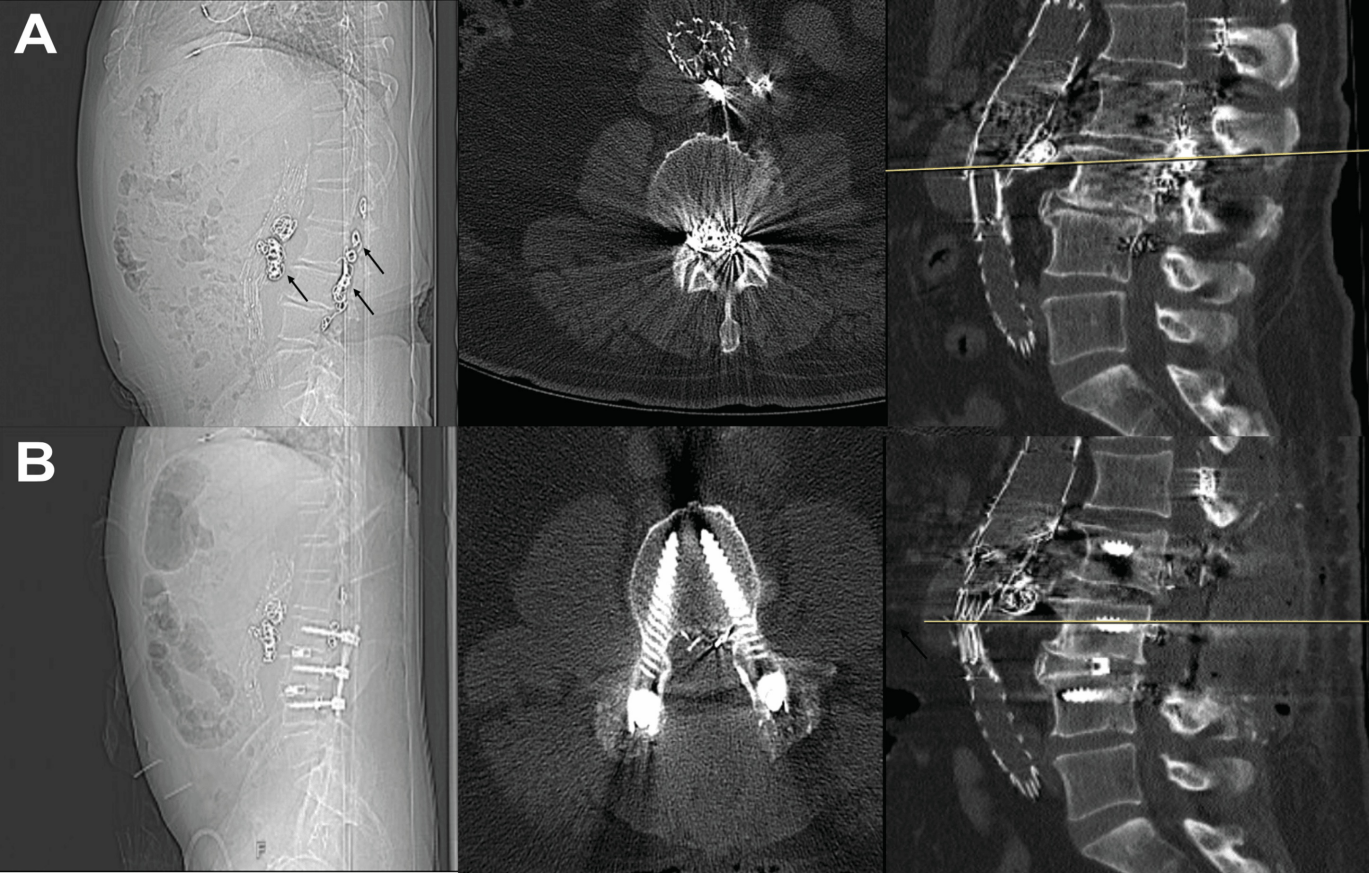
Preoperative (a) and postoperative (b) CT images of the lumbar spine Preoperative images show extravasated endovascular coils within the lumbar epidural plexus from L1 to L4 (black arrows, left panel). Stable post-interventional changes are also visible, consistent with prior fenestrated stent-grafting and secondary translumbar endovascular treatment of an AAA. On the sagittal images (right panel), yellow lines indicate the level of the axial slices shown in the middle panel for both preoperative (a) and postoperative (b) scans. AAA, abdominal aortic aneurysm
